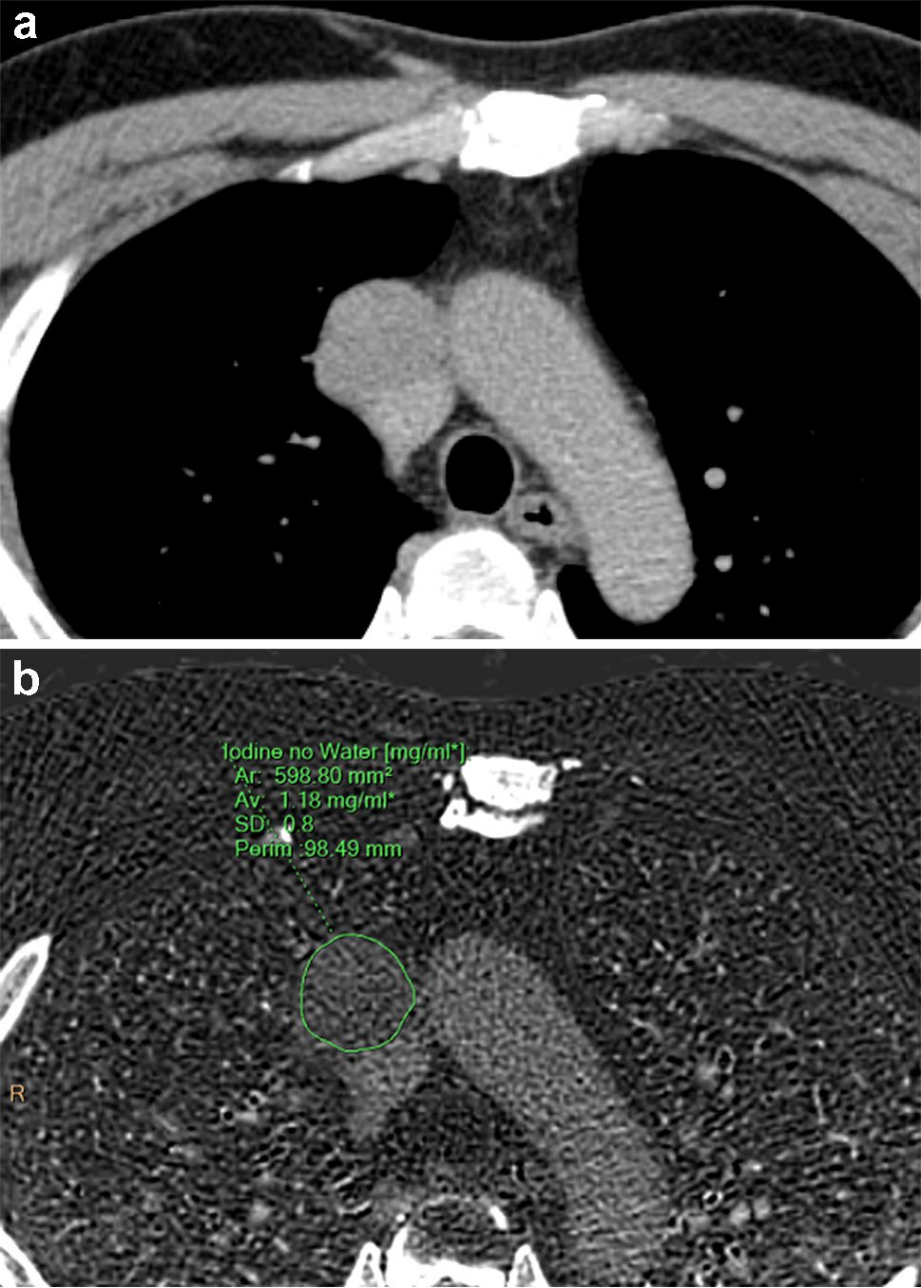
A 39-year-old male with low-risk thymoma (type AB). Contrast enhanced CT (a) shows a homogeneous, rounded, and well-defined tumor in the right anterior mediastinum. Iodine concentration during the equilibrium phase (b) and extracellular volume fraction are 1.18 mg/mL and 25.6%, respectively
